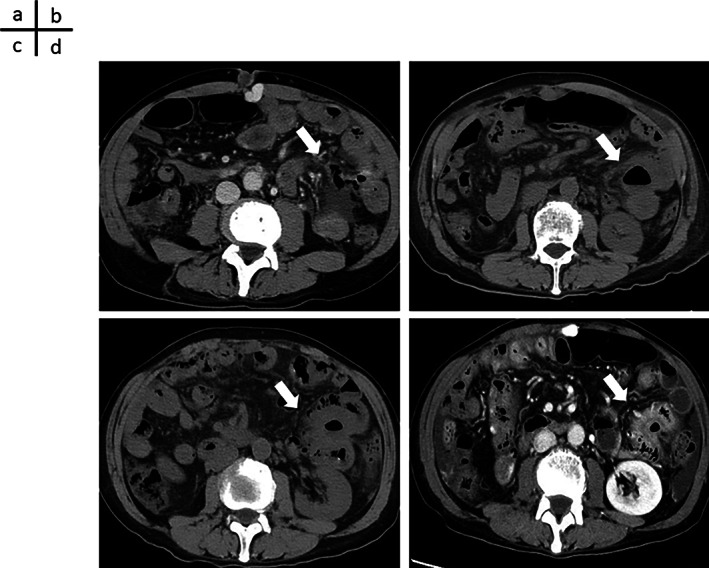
Changes in computed tomography (CT) images with the course of treatment (Arrows indicate perforated sites). (A) CT image on the third day of hospitalization. Significant edema of the jejunum and large intestine was observed. An air bubble in the mesentery and a jejunal perforation were noted. (B) CT image on the 22nd day of hospitalization (after two doses of infliximab). Improvement of gastrointestinal wall thickening and disappearance of free air was confirmed. (C) CT image on the 35th hospitalization day. Relapse of gastrointestinal wall thickening was noted. (D) CT image on the 56th day of hospitalization (after introduction of prednisolone). Improvement in gastrointestinal wall thickening was noted
